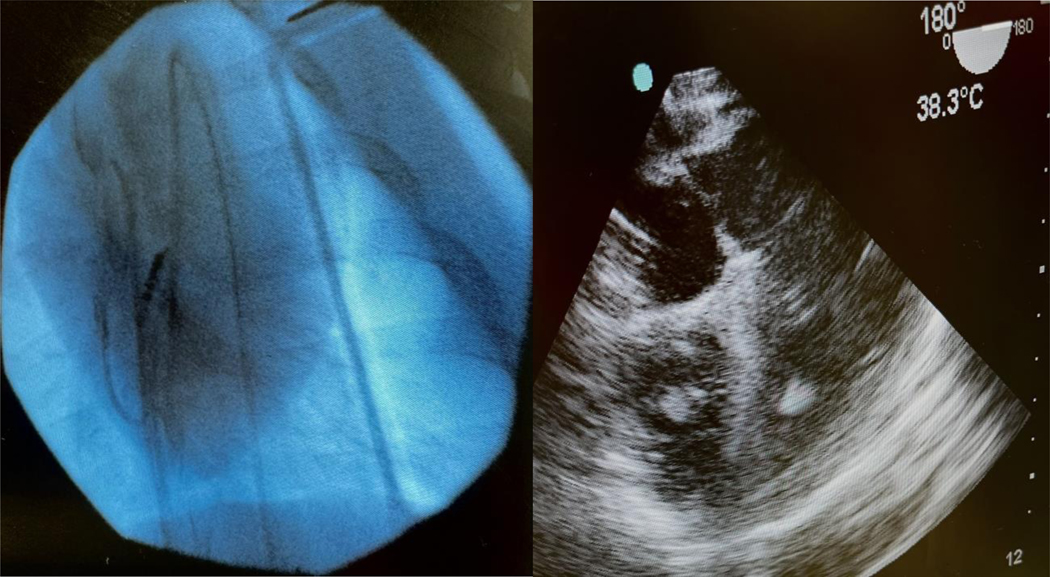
Biventricular pressure-volume loop system along with conductance catheters (top, CD Leycom, Hengelo, Netherlands), placement confirmation under fluoroscopy (bottom left) and transesophageal echocardiography (bottom right).
